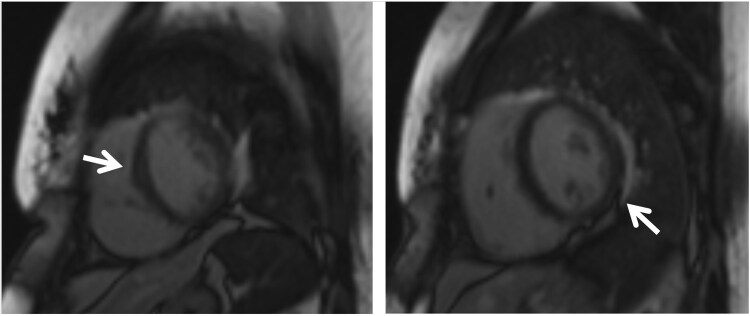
Cardiac magnetic resonance imaging showed patchy mid-wall to epicardial late gadolinium enhancement (arrow) at the apical anterior, basal anteroseptal/inferolateral, anterolateral, inferoseptal, mid-inferoseptal, mid-inferior, and apex, which was suggestive of non-ischaemic causes including infiltrative heart disease such as cardiac sarcoidosis or myocarditis. No CMR evidence to suggest arrhythmogenic right ventricular cardiomyopathy was seen. There was reduced ejection fraction of 26.3% and wall motions of all segments were normal.
